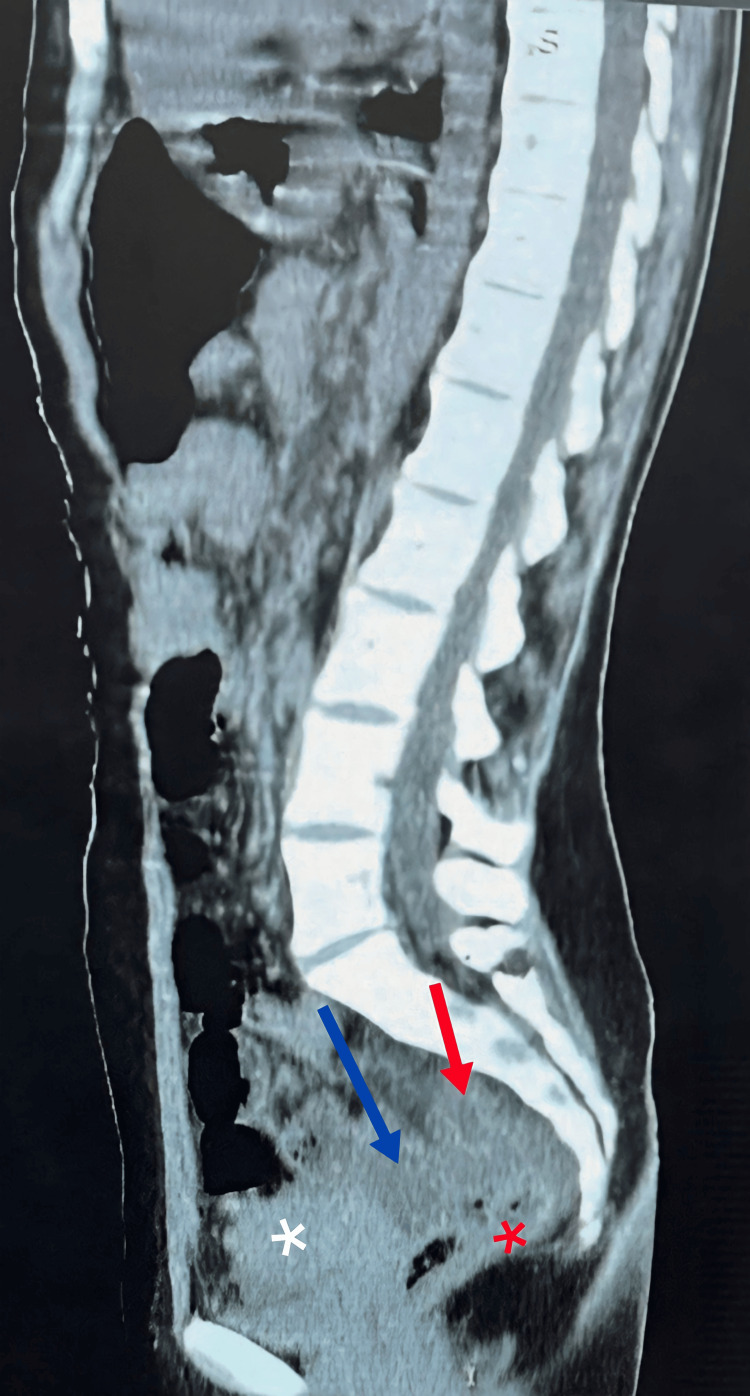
Abdominopelvic CT scan (sagittal view) shows the suspected herniated bowel segment (red arrow) between the uterus and rectum in addition to the presence of pelvic free fluid (blue arrow). Uterus (white asterisk), rectum (red asterisk)
